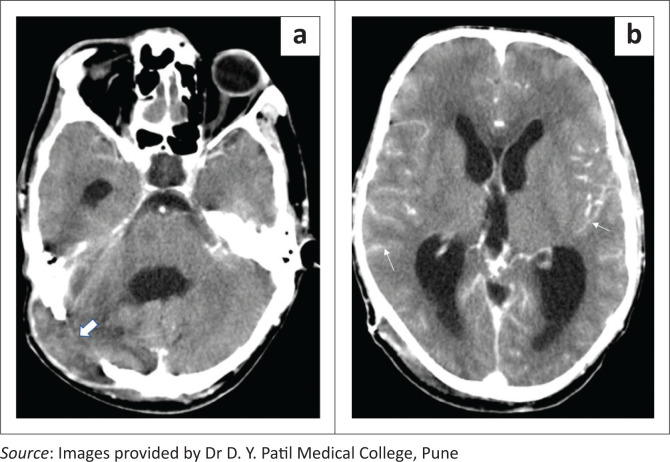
Meningitis. Axial post-contrast soft tissue window CT image in a patient with a right occipital craniectomy (a, thick white arrow) indicates extensive leptomeningeal enhancement (b, thin white arrows). Herniation of the cerebellar parenchyma is also seen at the occipital craniectomy site.
